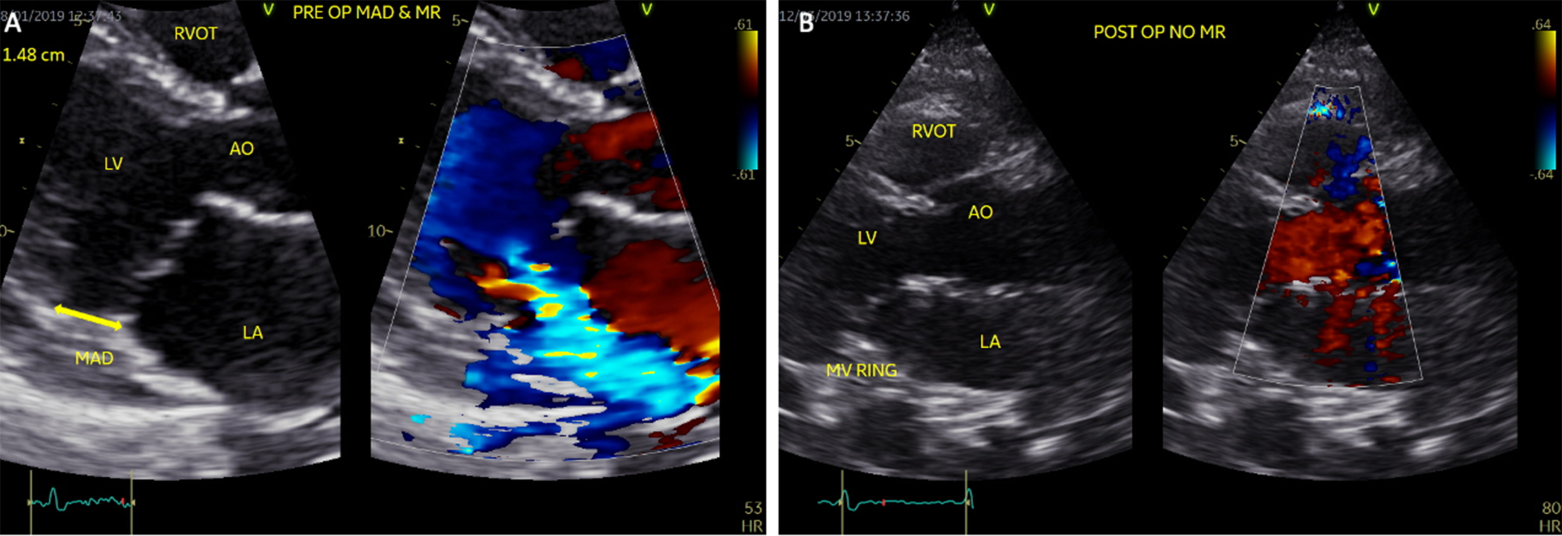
Transthoracic echocardiogram of a 60-year-old female patient.
